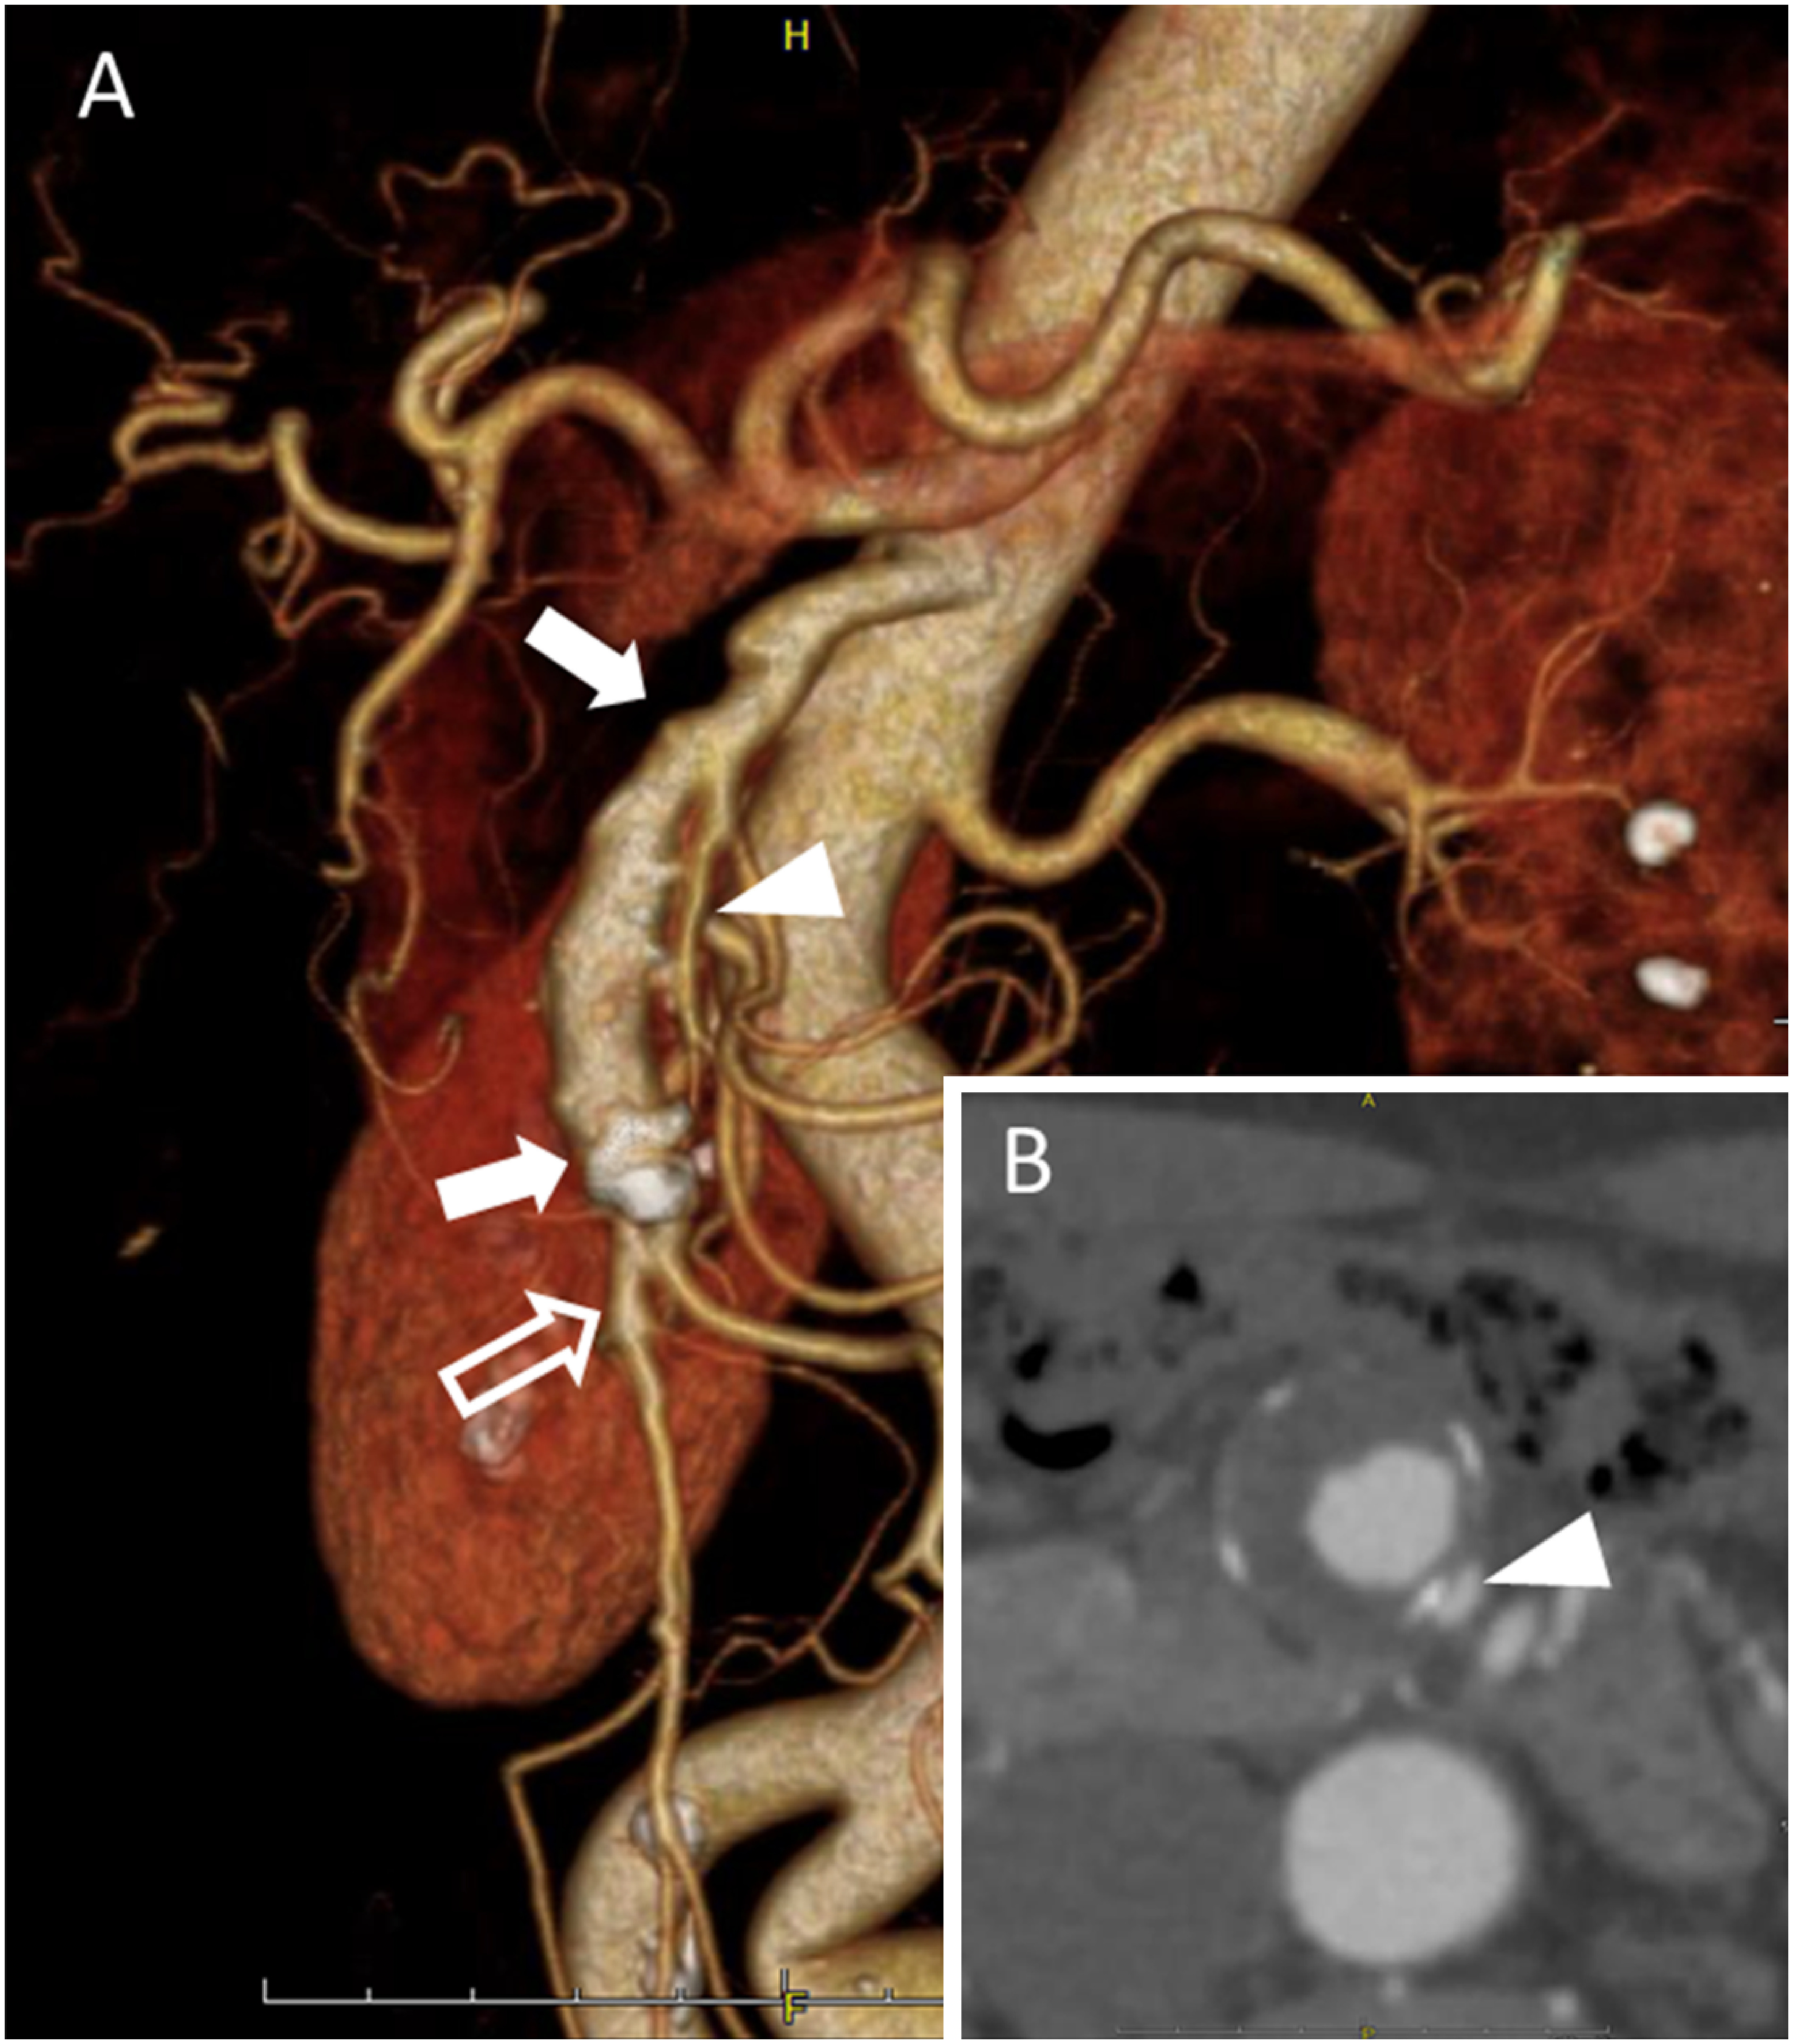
Fig. 1 Preoperative computed tomography angiography (A, a volume-rendered image; B, an axial view). The superior mesenteric artery aneurysm had a diameter of 25 mm and was located 20 mm distal to the origin of the superior mesenteric artery. The aneurysm and dissection longitudinally extended 3 cm distal to the origin of the aneurysm (➡). The true lumen of the aneurysm (▼) gave rise to middle colic artery, three jejunal arteries, and right colic artery from the proximal end. The ileocolic artery (⇒) originated from the superior mesenteric artery just distal to the end of the aneurysm.
